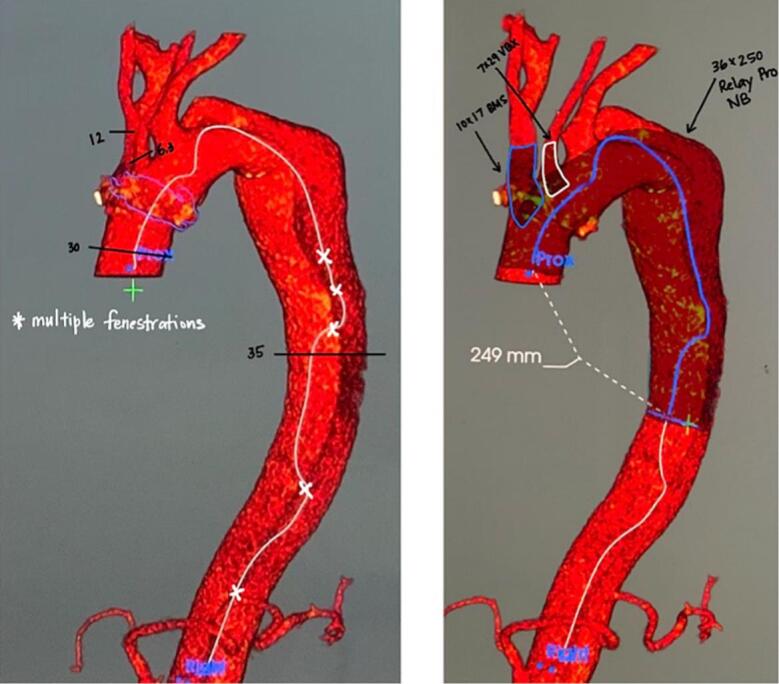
3D CT of thoracic aorta. * Indicates tear in medial layer of the vessel.
